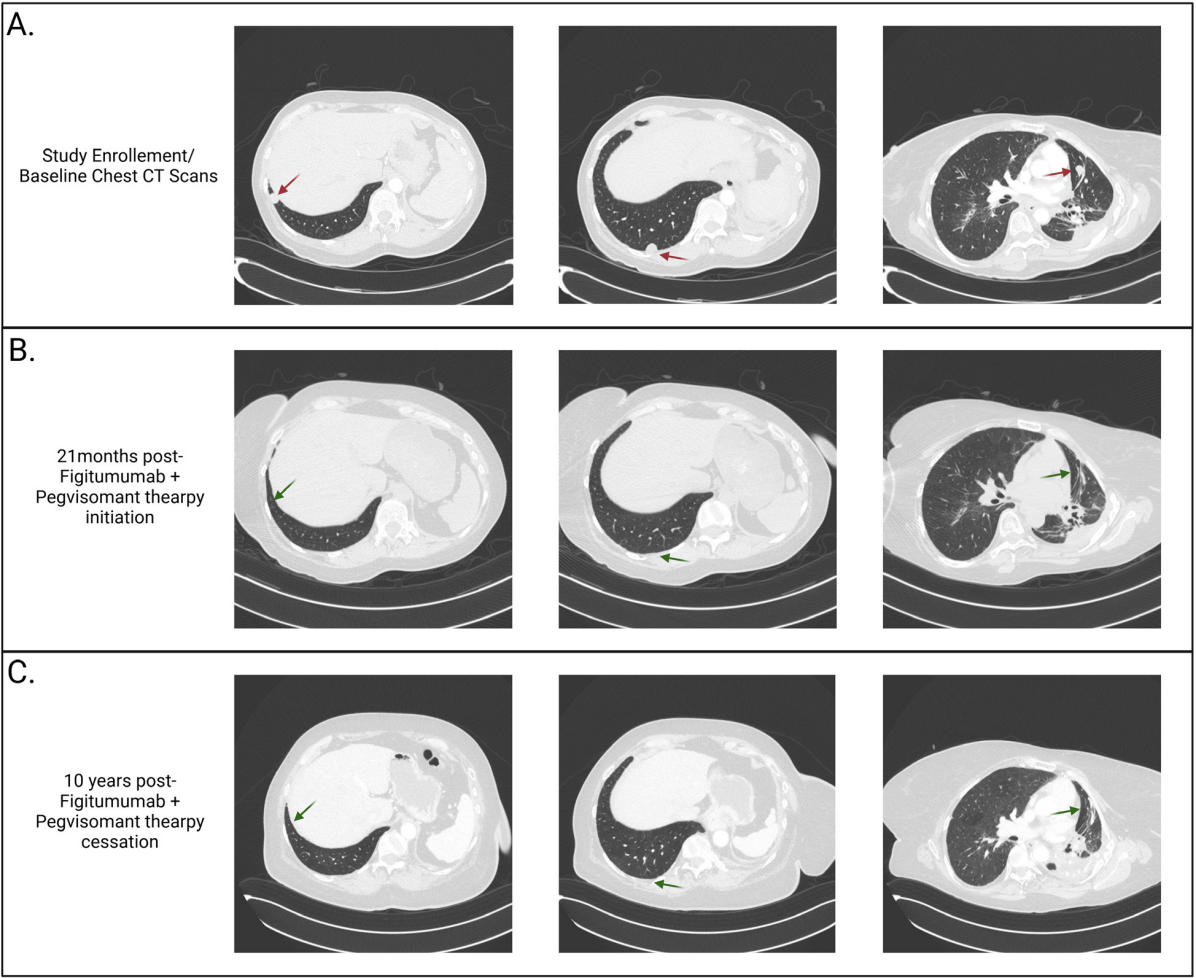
Computerized tomography (CT) scans. (A) Baseline CT scans of the lung demonstrating three intraparenchymal lung nodules (1.3 cm in diameter in the posterior and lateral right lower lobe, 1.4 cm in the subpleural right lower lobe, and 1.4 cm in the left mid-lung lobe) and post-radiation fibrosis (due to previous lines of therapy) prior to enrollment in the NCT00976508 clinical trial. (B) CT scans taken twenty-one months following clinical trial enrollment and two months following the completion of figitumumab and pegvisomant therapy, demonstrating complete resolution of the three lung nodules. (C) CT scans obtained ten years after discontinuing figitumumab and pegvisomant therapy, demonstrating sustained resolution of the lung nodules.
